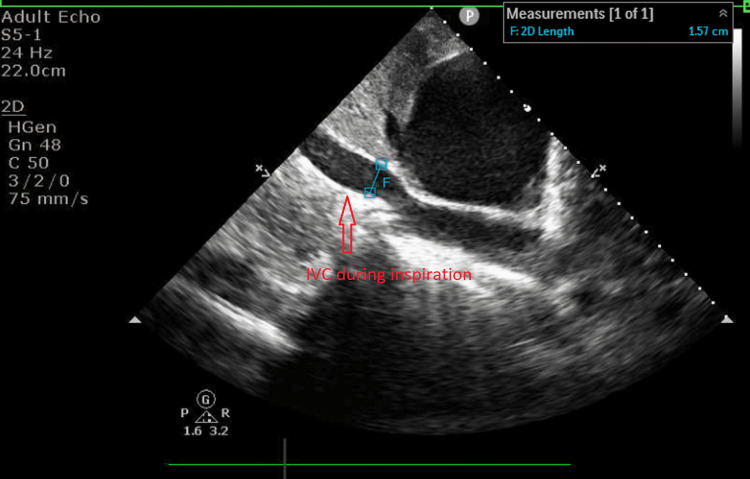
Transthoracic echocardiography (subcostal view) The size of the inferior vena cava was 1.57 cm during inspiration. IVC, inferior vena cava.
